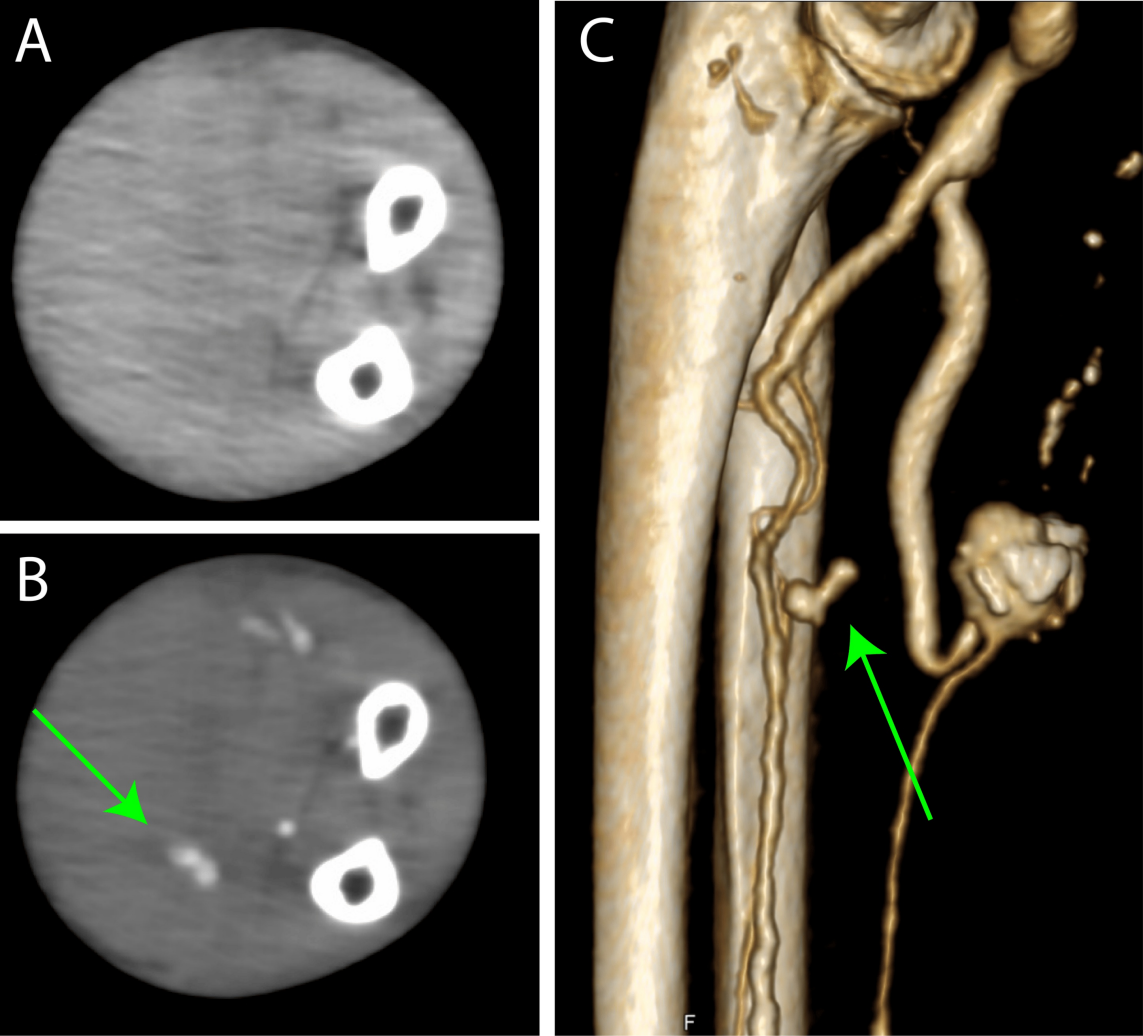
Preoperative images at the age of 72 years old. A computed tomography (CT) image of the left forearm. (A) Plain CT: Swelling of the left forearm is observed. (B) Venous phase: Venous bleeding is observed (green arrow). (C) Volume rendering image: Venous bleeding is observed (green arrow).
